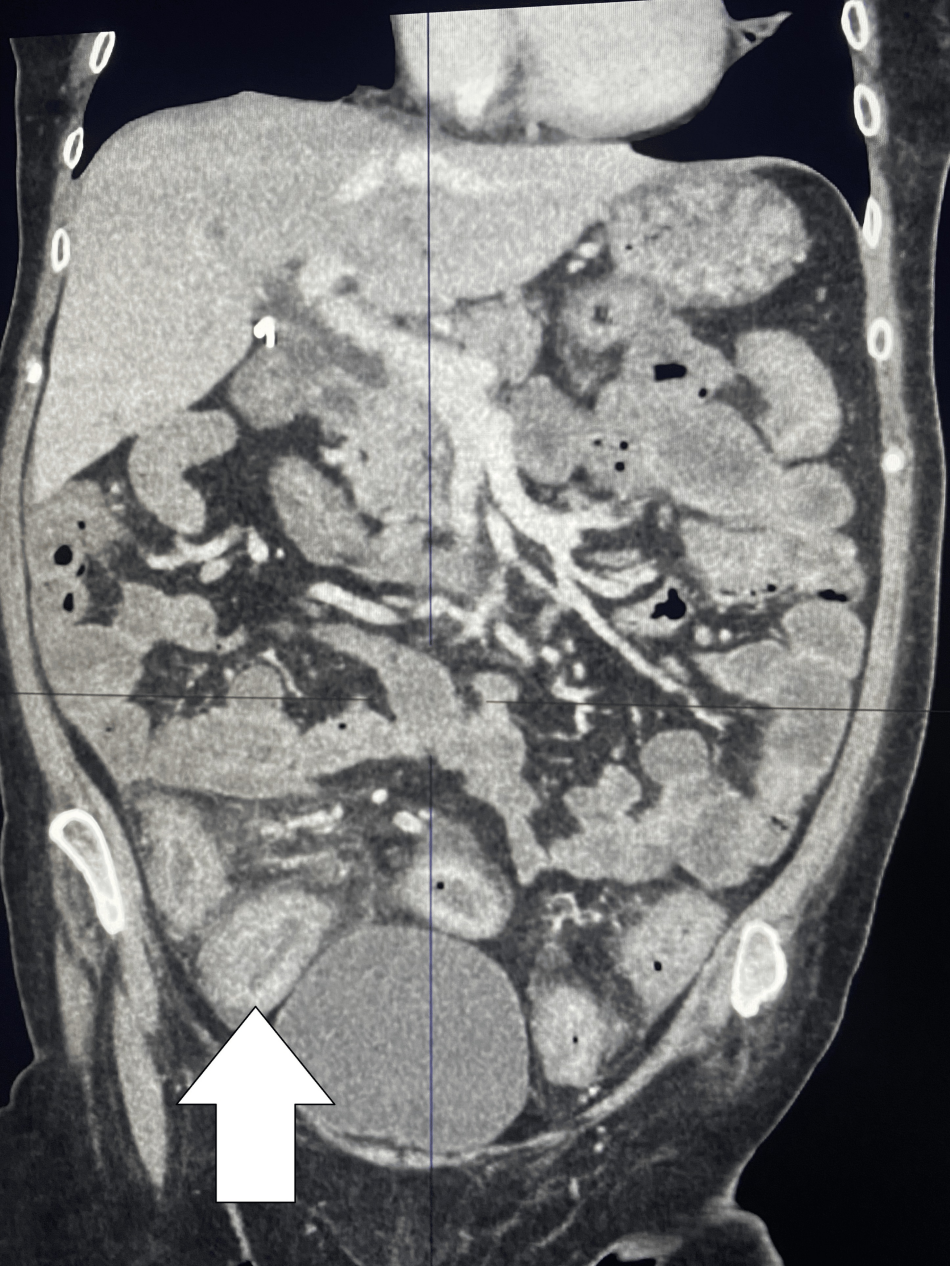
CT Abdomen and Pelvis From March 2025 Showing Active Colitis Contrast-enhanced coronal CT image demonstrating diffuse colonic wall thickening and edema. The white arrow highlights a segment of notably thickened bowel.
